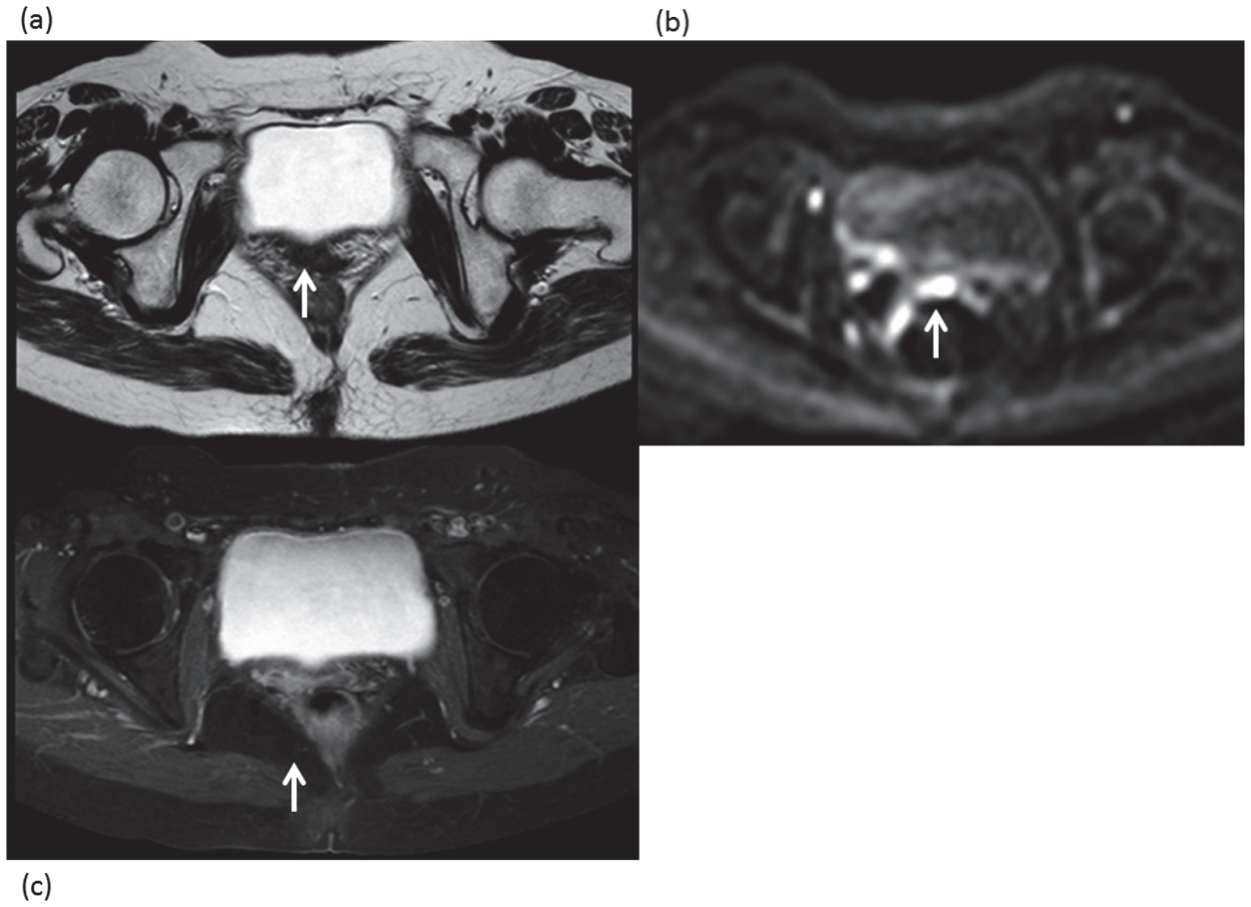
A 54-year-old woman with a locally recurrent tumor 10 months after surgery for uterine cervical cancer. (a) Axial T2-weighted MRI shows a small, slightly hyperintense area in the vaginal vault (arrow). This equivocal finding for local recurrence was assigned a score of 3 for protocol A. (b) Axial DWI shows a focal hyperintense area in the posterior urinary bladder (arrow), corresponding to the area seen in (a), suggesting local recurrence. Protocol B was assigned a score of 5 for local recurrence. (c) Axial contrast-enhanced MRI shows abnormal enhancement as the same site (arrow), suggesting local recurrence. The score assigned for protocol C was 5. Biopsy confirmed the presence of tumor recurrence.
